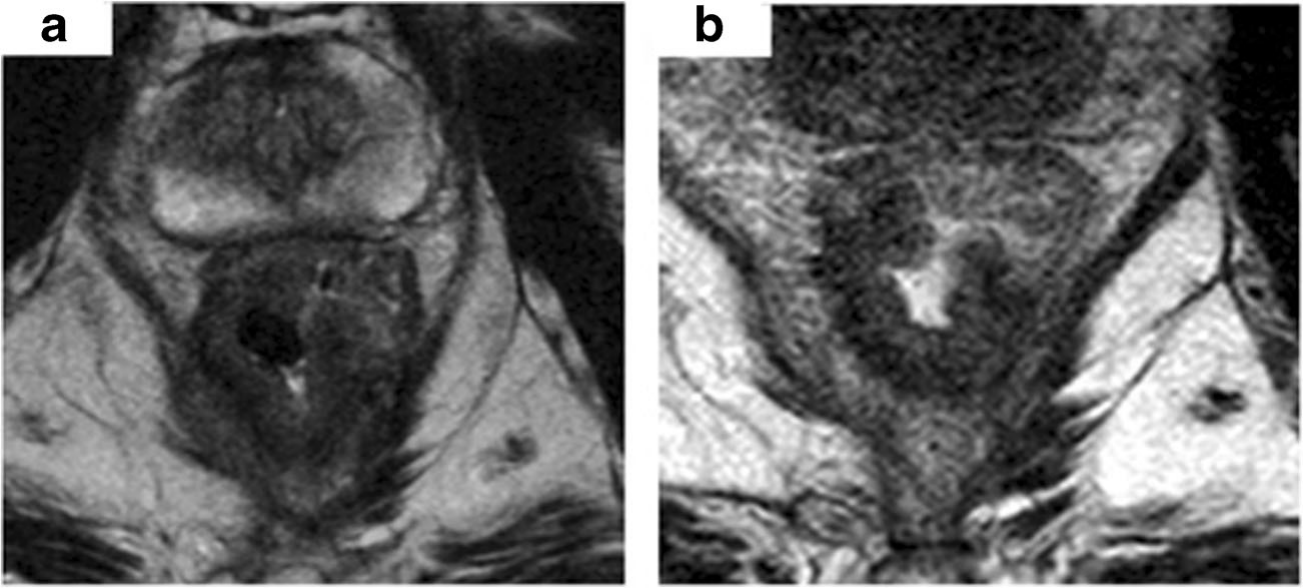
Low rectal tumour before treatment, infiltrating the rectal wall at 5–10 o’clock position with evidence of spread beyond the muscularis and spread into the intersphincteric plane, with invasion of the right levator (a). On post CRT MRI, the treated tumour demonstrates intermediate signal predominantly suggestive of macroscopic residual disease—mrTRG4 (b)
